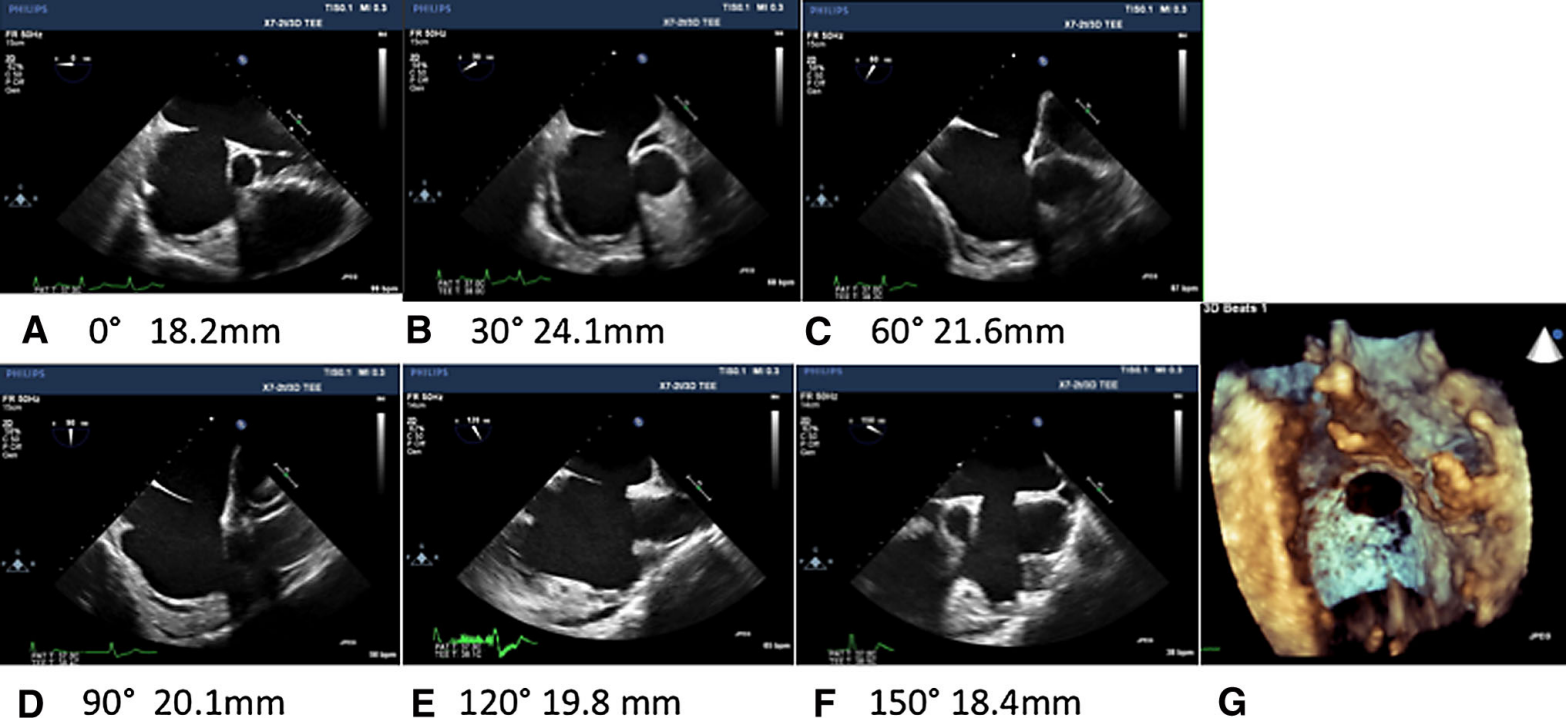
Transesophageal echocardiography of the atrial septal defect. Two-dimensional images and defect diameters each at 30° (a–f) and a three-dimensional right atrial en-face view image (g) are shown. The maximum defect diameter was 24.1 mm at 30°, and the minimum defect diameter was 18.2 mm at 0°. The aortic rim was deficient at 30°–60°, and the superior rim was deficient at 60°–90°. The posterior rim was not floppy and was sufficiently long. Septal malalignment was observed at 60° and 90°
